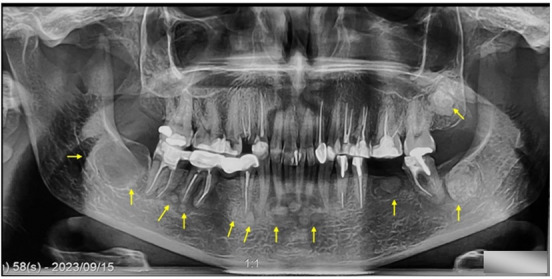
Panoramic radiograph showing the cystic lesion with the cemento-osseous dysplasia lesions in the mandible and maxilla
